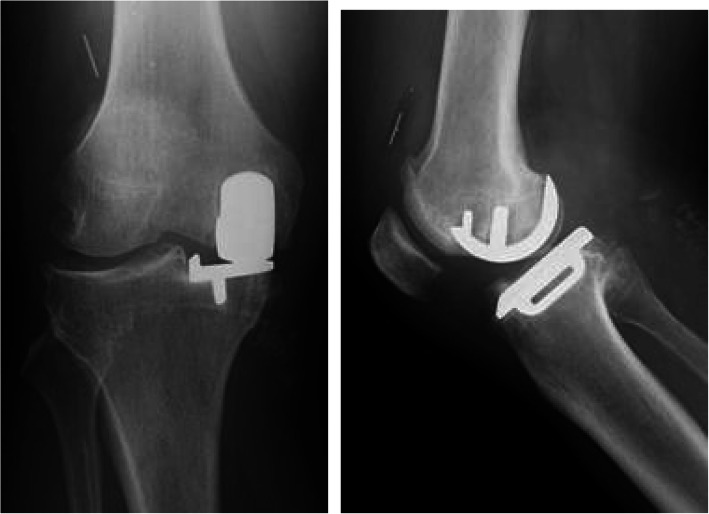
Postoperative radiographs of case 2 at 6 months showing bearing dislocation
